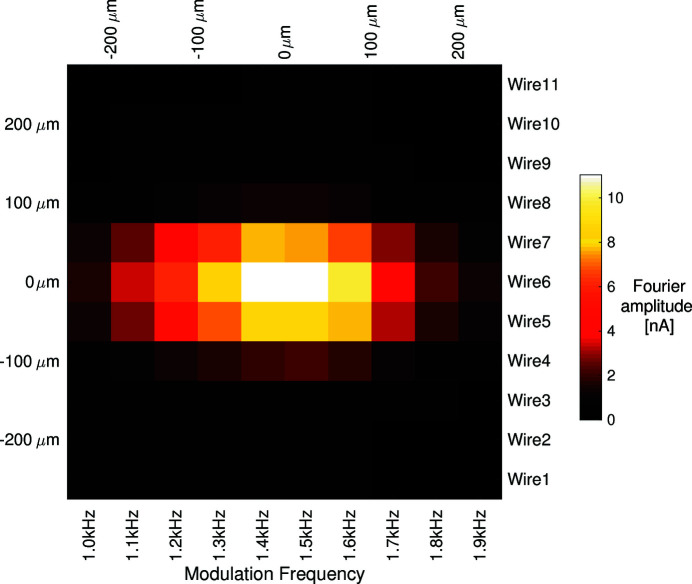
The reconstructed image of the X-ray beam footprint on the detector.
